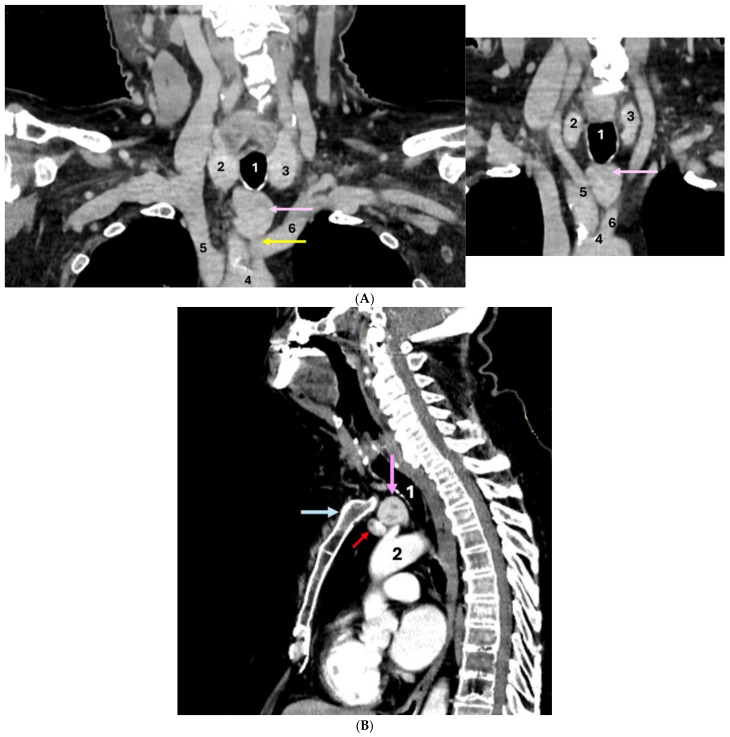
Contrast-enhanced CT scan showed: (A) Coronal plane (two different sections): 1—trachea; 2—right thyroid lobe; 3—left thyroid lobe; 4—ascending aorta; 5—brachiocephalic trunk; 6—left common carotid artery; ectopic thyroid (pink arrow); arterial supply (yellow arrow) for the ectopic thyroid, originating from the left common carotid artery. (B) Sagittal plane: 1—trachea; 2—ascending aorta; venous supply (red arrow) for ectopic thyroid, originating from the left brachiocephalic venous trunk; ectopic thyroid (pink arrow); manubrium (blue arrow).
